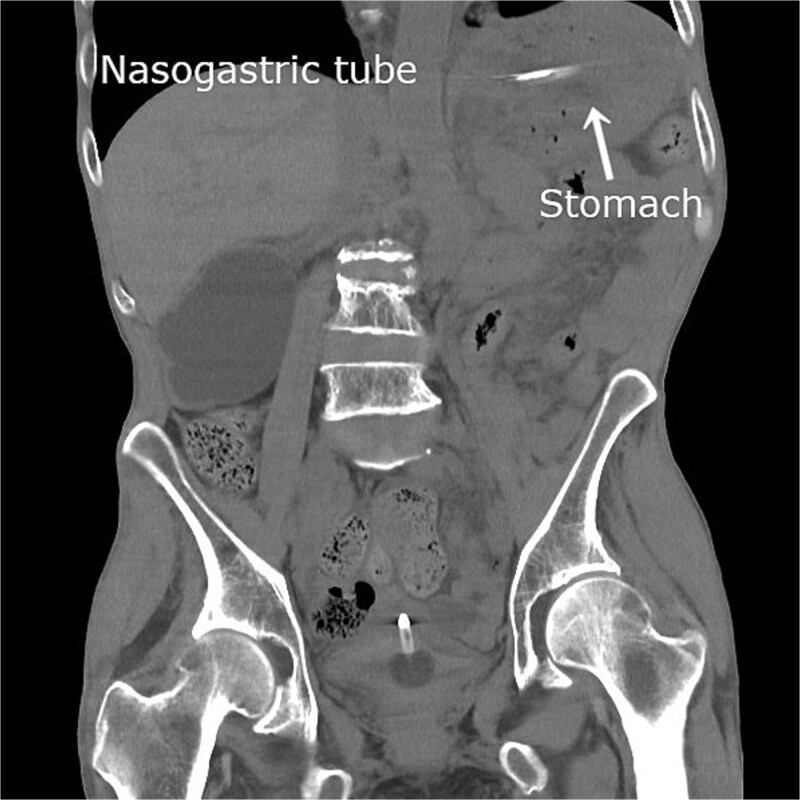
Follow-up plain CT obtained on the day after admission revealing gastric dilatation resolution following nasogastric decompression. The stomach has returned to its normal anatomical position, indicating spontaneous hernia reduction.
